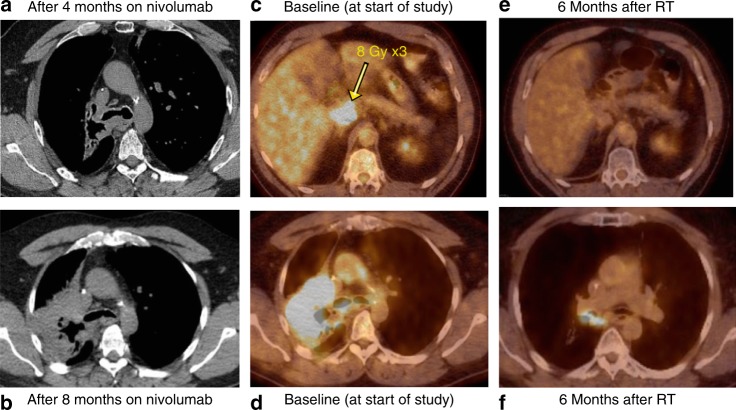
Images for responder with non-small cell lung cancer. Patient 15 was diagnosed with non-small cell lung cancer metastatic to the bone and was given carboplatin/paclitaxel x 6 cycles with a good response and then given palliative radiation to the right lung mass (37.5 Gy). He developed a mass in the ileum and received gemcitabine/navelbine but progressed. He received nivolumab to which he had a good response initially. (a) Chest CT scan after 4 months on nivolumab. CT scan 2 months later showed progression of disease in the chest. CT scan done 2 months after this showed further progression of disease (b). At this time, nivolumab was discontinued, and he was enrolled on our pembrolizumab HFRT study. He underwent a planning FDG PET/CT scan that established his baseline disease status (c, d). He received pembrolizumab and then radiation (8 Gy × 3) to an abdominal mass (c) followed by continued pembrolizumab. A repeat PET/CT scan was performed 6 months after radiation (e, f) showing response in non-irradiated lesions which had previously progressed on PD-1 blockade prior to study entry
